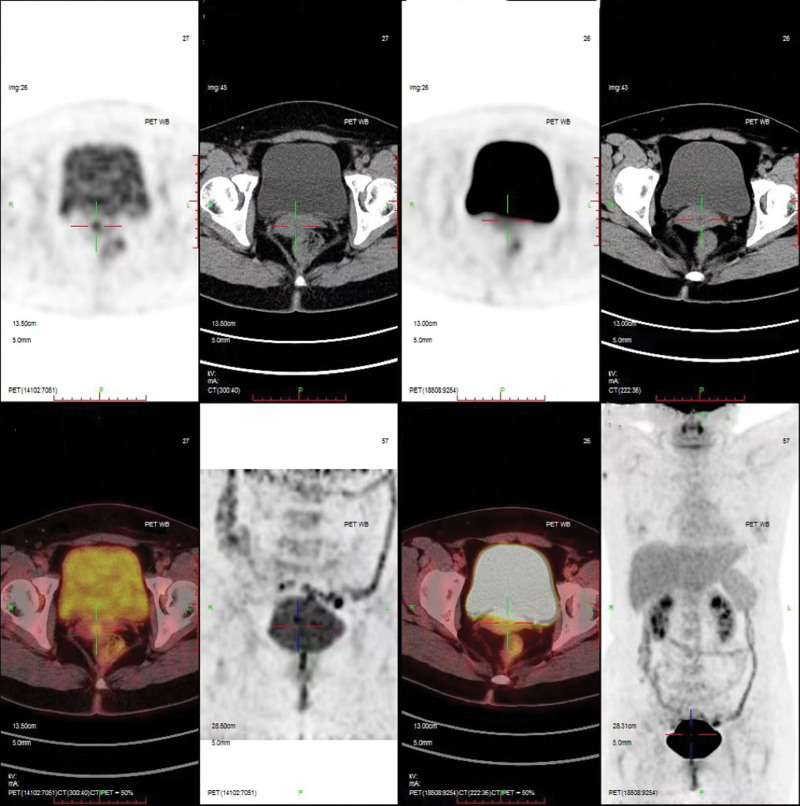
The positron emission tomography (PET) revealed an increased ^18^F-fluorodeoxyglucose (^18^F-FDG) metabolism in the local cervix (line marking).
